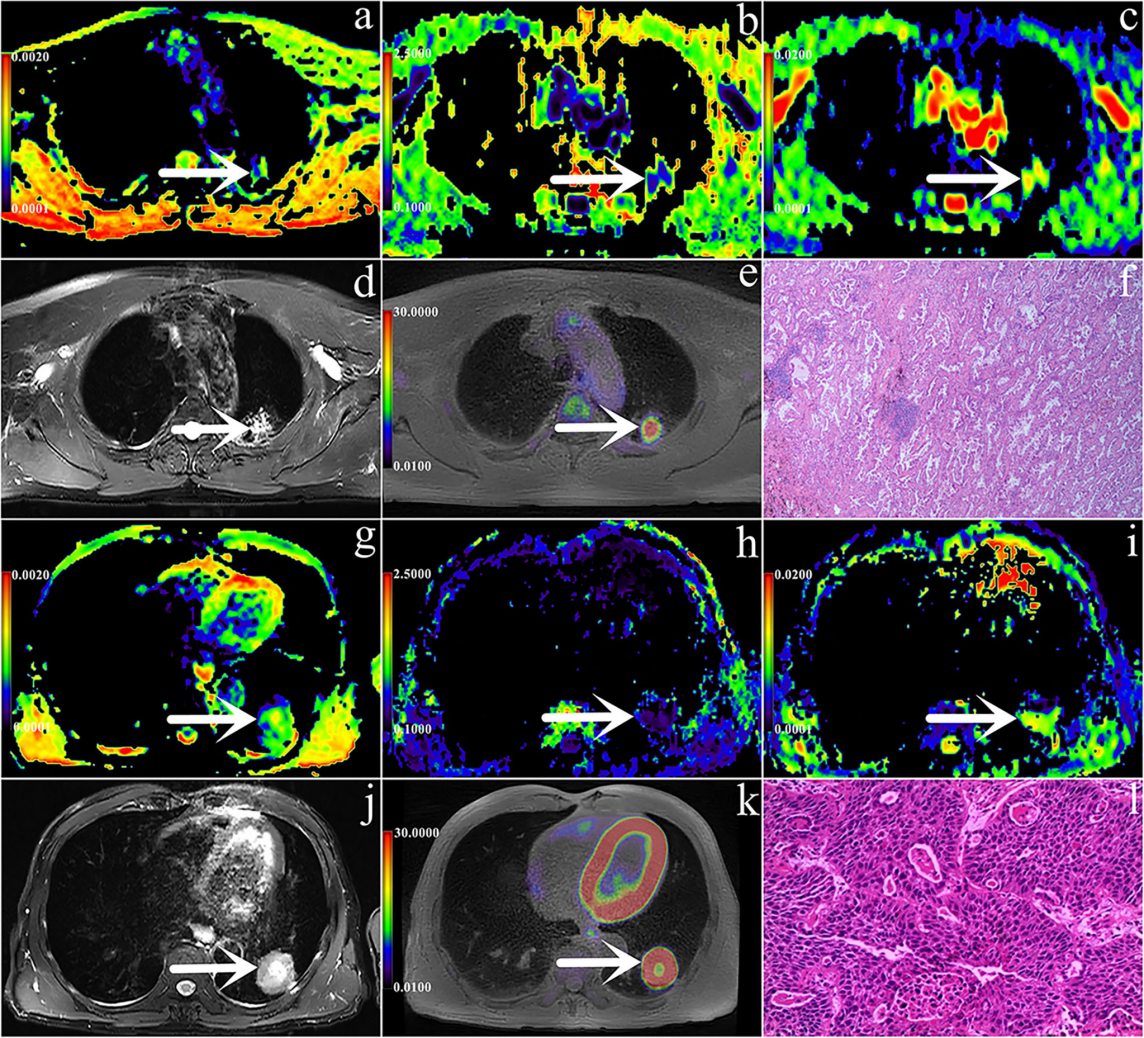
(A–L) are PET/MR images and pathological images. (A–F) Male, 56 years, with staging IIB, AC (shown by white arrows), Ki-67 is 30%, ADC = 1.505 × 10^-3^mm^2^/s, MK = 0.652, MD = 2.768 × 10^-3^mm^2^/s, SUVmax = 5.08 g/cm^3^, MTV = 2.44 cm^3^, TLG = 7.28 g; g-l Male, 58 years, with staging IIIB, SCC(shown by white arrows), Ki-67 is 70%, ADC = 1.068 × 10^-3^mm^2^/s, MK = 0.291, MD = 2.622 × 10^-3^mm^2^/s, SUVmax =11.84 g/cm^3^, MTV = 12.248 cm^3^, TLG = 83.408 g. In these images, a/g are ADC pseudo-colored maps, b/h are MK pseudo-colored maps, c/i are MD pseudo-colored maps, d/j are T2WI images, e/k are PET images and f/l are pathological images (magnification=100).
